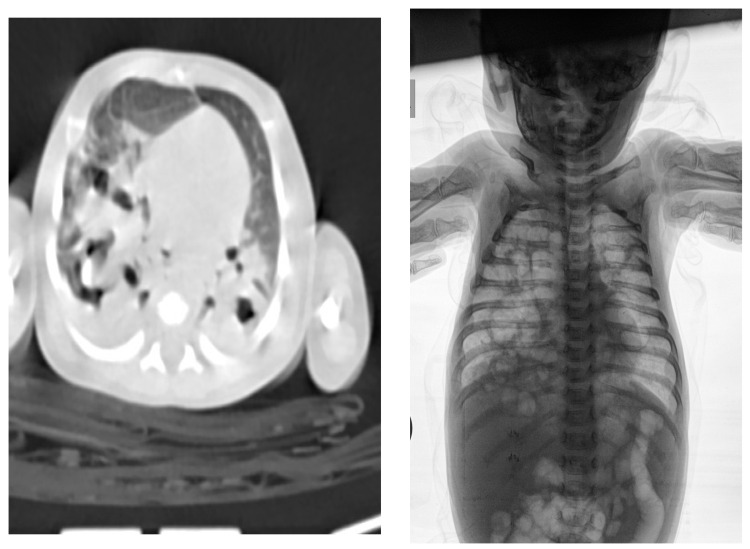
MSCT and X-ray of thoracic in neonate 1 indicate extensive, confluence, zones of lung parenchyma consolidation, on both sides, with signs of necrosis and formed thick wall cavities. The multislice computed tomography (MSCT) of the thoracic on the 17th day of hospitalization and X-ray of the thoracic on the 39th day of hospitalization shows cystic formations in the lungs. The MSCT finding on 17th day: The examination was performed natively and postcontrast, with axial sections 0.8 mm thick, with subsequent reconstructions in MPR and VRT. On both sides, diffuse in the lung parenchyma, large confluent zones of lung parenchyma consolidation can be seen, with hypodense zones of necrosis (density about 30 HU). Within the described consolidation zones, numerous thick-walled caverns can be seen, some of which communicate with each other, up to 13 mm in diameter. The anterior segments of the upper and lower lobes and the medial lingula segment of the right lung, as well as the anterior segment of the lower left lobe, are enveloped in aerations with smaller circular zones of consolidation. In the pleural cavities, on both sides, no free fluid is observed. The trachea, main, lobar, and segmental bronchi are preserved lumen and arborization. No significant mediastinal lymphadenopathy was observed. No pathological changes were seen in the bone structures of the chest wall shown. No pathological changes were seen in the sections through the upper abdomen. The X-ray finding on 39th day of hospitalization: Bilateral, diffuse in the pulmonary parenchyma of multiple oval cavitation zones with different wall thicknesses, which probably correspond to legionnaires’ disease. On the right are possible aero-liquid levels. Right hemidiaphragm of obscure contours.
